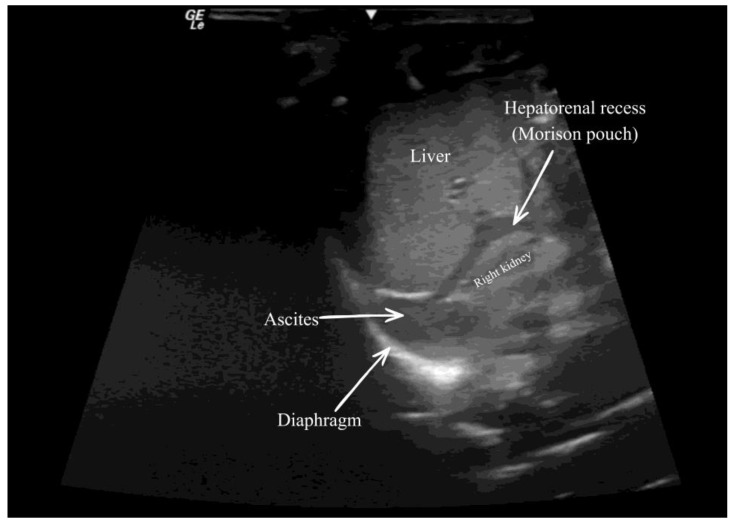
Right upper quadrant (perihepatic) abdominal ultrasound demonstrating free anechoic intraperitoneal fluid (ascites) (arrow) adjacent to the liver and within the hepatorenal recess (Morison pouch) (arrow). The right kidney and diaphragm are labeled for anatomic orientation.
